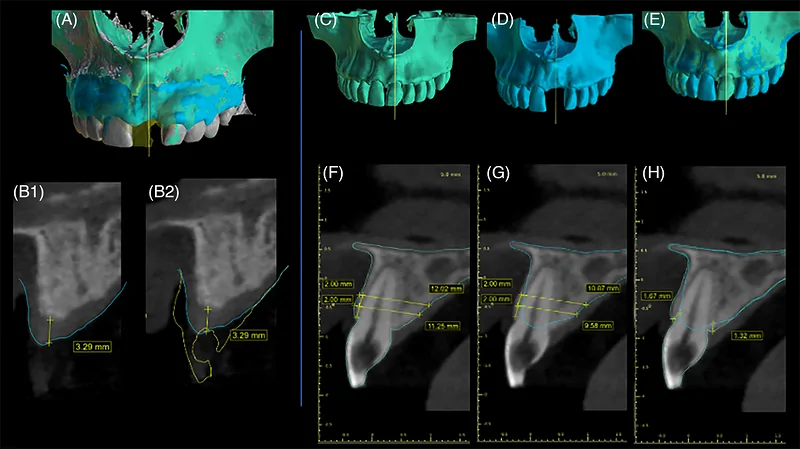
(A) Mid‐sagittal reference slice over 3D CBCT reconstructions superimposed with intraoral scans. (B) CBCT and intraoral scans with soft tissue mid‐crestal thickness. (B1) CBCT and intraoral scan 4 months after extraction; (B2) additional superimposition of baseline intraoral scan, horizontal and vertical differences are seen between intraoral scan. (C–E) Segmented CBCT as bone surfaces. (C) Left, Baseline bone surface. (D) Four months after extraction bone surface. (E) Aligned bone surfaces. (F–H) Bone surfaces outlines superimposed to the baseline CBCT mid‐sagittal slice. Vertical measurements of 2 mm from the buccal crest (2 and 4 mm) are shown for horizontal measurements references. (F) Baseline CBCT and baseline bone surface superimposed, horizontal measurements made following 2 and 4 mm references. (G) Baseline CBCT and 4 months after extraction bone surface superimposed with horizontal measurements. (H) Baseline CBCT, baseline and 4 months after extraction bone surfaces superimposed. Buccal and palatal vertical measurements of bone surface differences.
